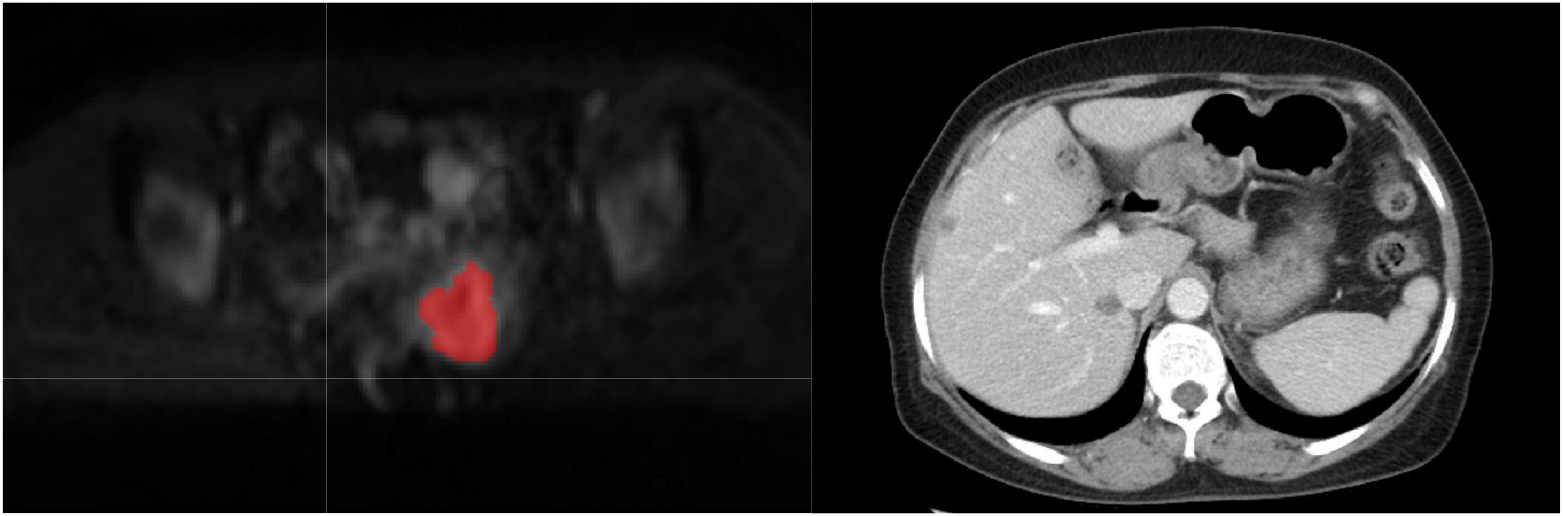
The red portion in the DWI image represents the outlined region of interest (ROI). This 50-year-old cervical cancer patient showed liver metastasis in the follow-up enhanced CT examination.
